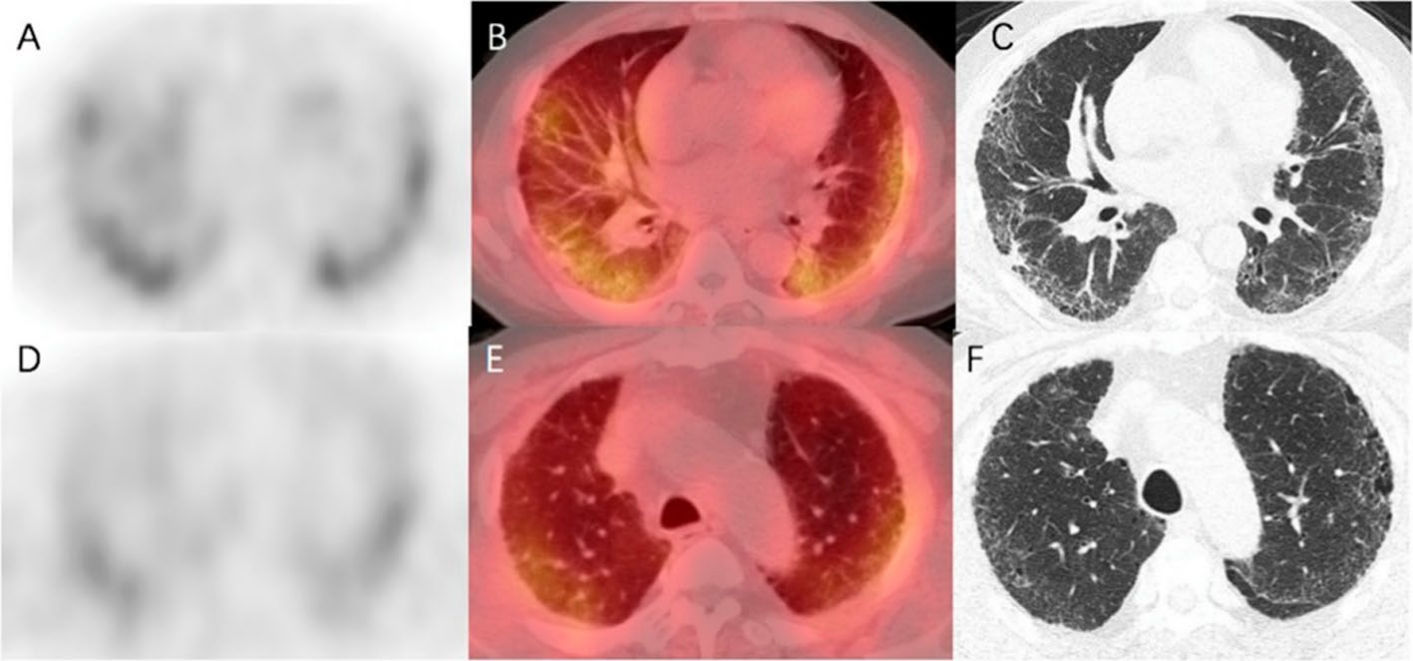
Fused axial SPECT/CT images in a patient affected by idiopathic pulmonary fibrosis. Upper row: corresponding emissive A, fused B and transmissive C slices of the lower left and right pulmonary lobes; note the intense tracer incorporation. Lower row: lower tracer incorporation can be observed in the corresponding emissive D, fused E and transmissive F slices of the middle-upper region of the lungs. Reprinted from (Liu et al. 2023b), under a Creative Commons Attribution 4.0 International License (http://creativecommons.org/licenses/by/4.0/). No changes were made
